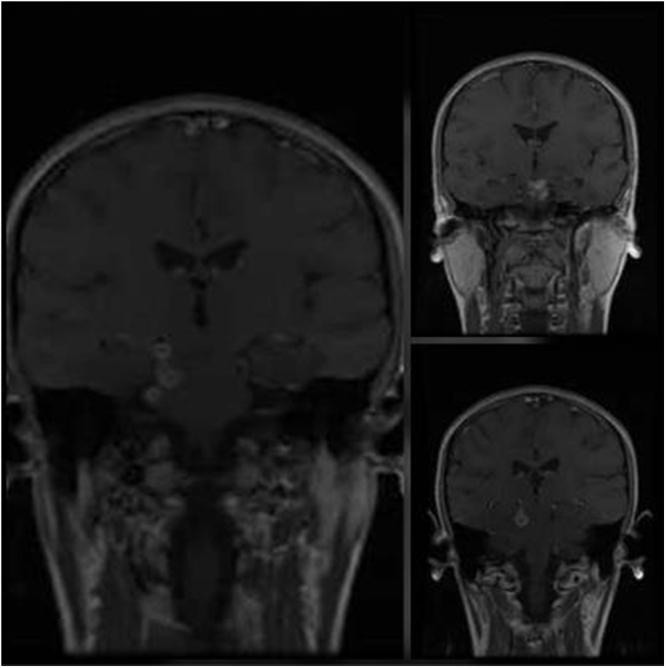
Coronal T1-weighted MRI brain post-gadolinium demonstrating multiple ring-enhancing lesions.
